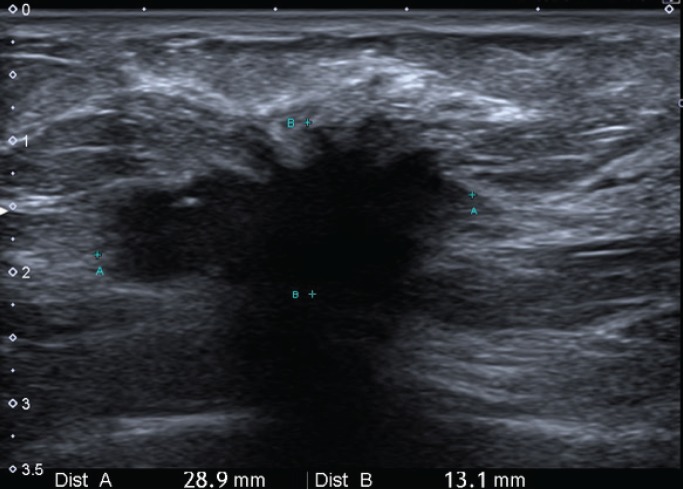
Ultrasonic left breast showing a hypoechogenic-spiculated mass with the acoustic shadow of 29 × 24 × 14 mm3 in correspondence with the mammographic image.
